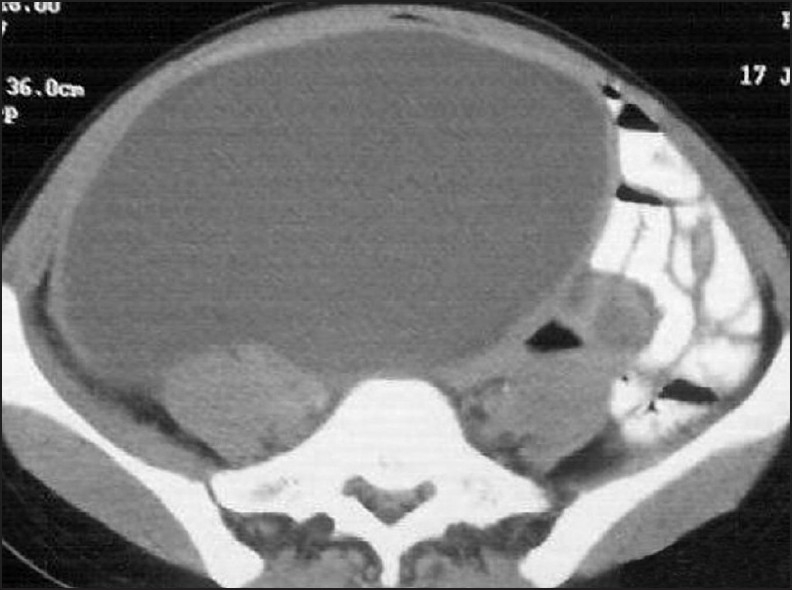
Computed tomography scan showing large cyst in the abdomen arising from the right adenexa
